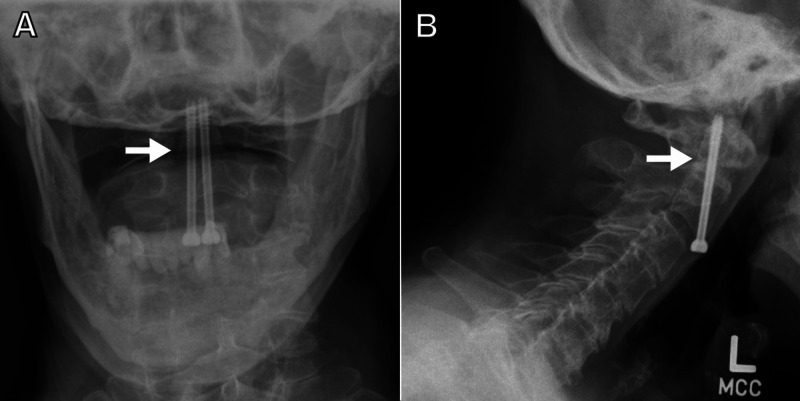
Three-month follow-up X-rays confirm healing of the fracture (A) AP and (B) lateral views of the cervical spine demonstrating union across former fracture site (arrows) AP: anteroposterior
